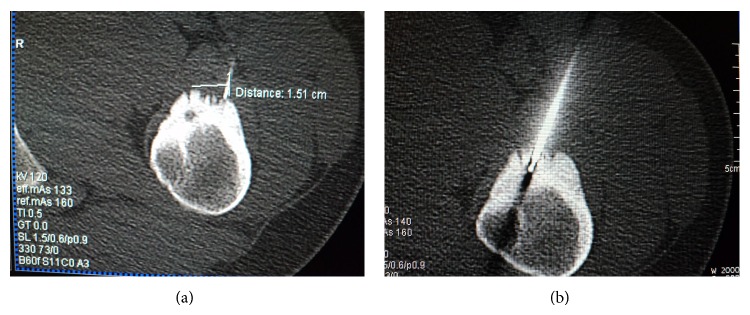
Spot CT images are obtained to confirm appropriate positioning.
